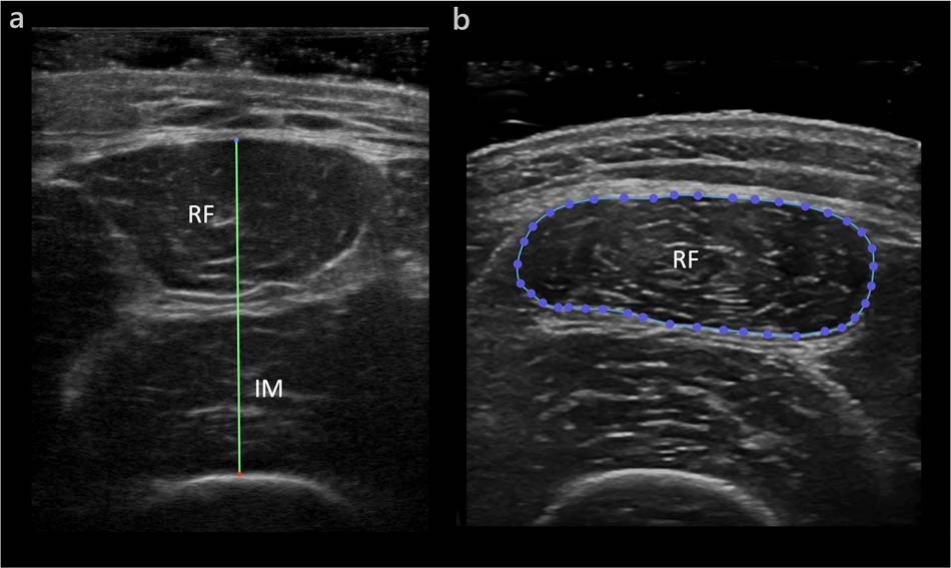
Example of ultrasound image analysis. (a) Quadriceps muscle layer thickness. (b) Cross-sectional area of the rectus femoris muscle. *RF* rectus femoris, *IM* intermedius. Adapted by Schieffelers DR *et al*. [61]. Copyright 2023 Elsevier
